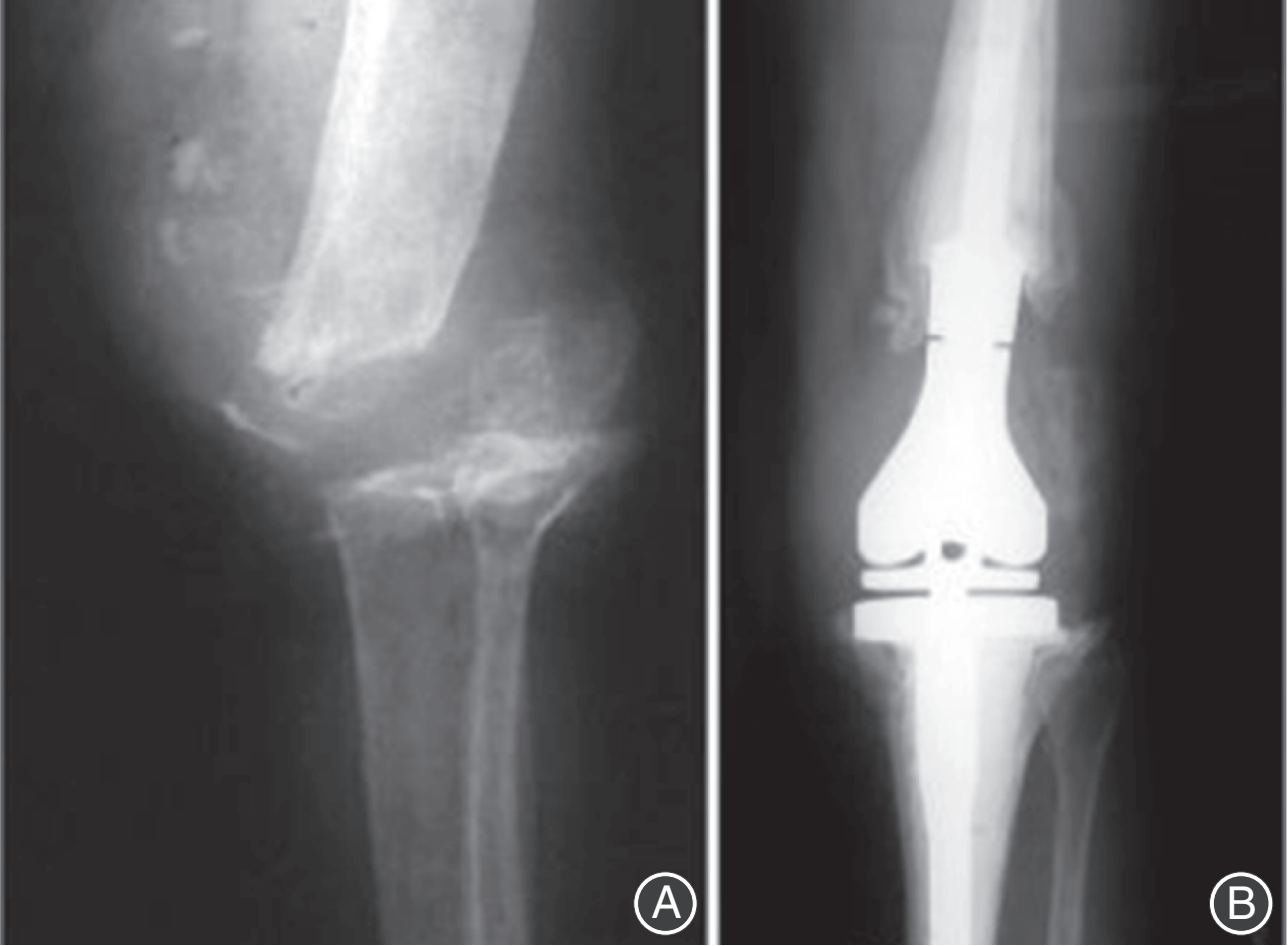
(A) Preoperative radiograph showing complex bone defects. (B) Postoperative anteroposterior radiograph showing treatment with a megaprosthesis with a rotating hinge device severe bone defects.
